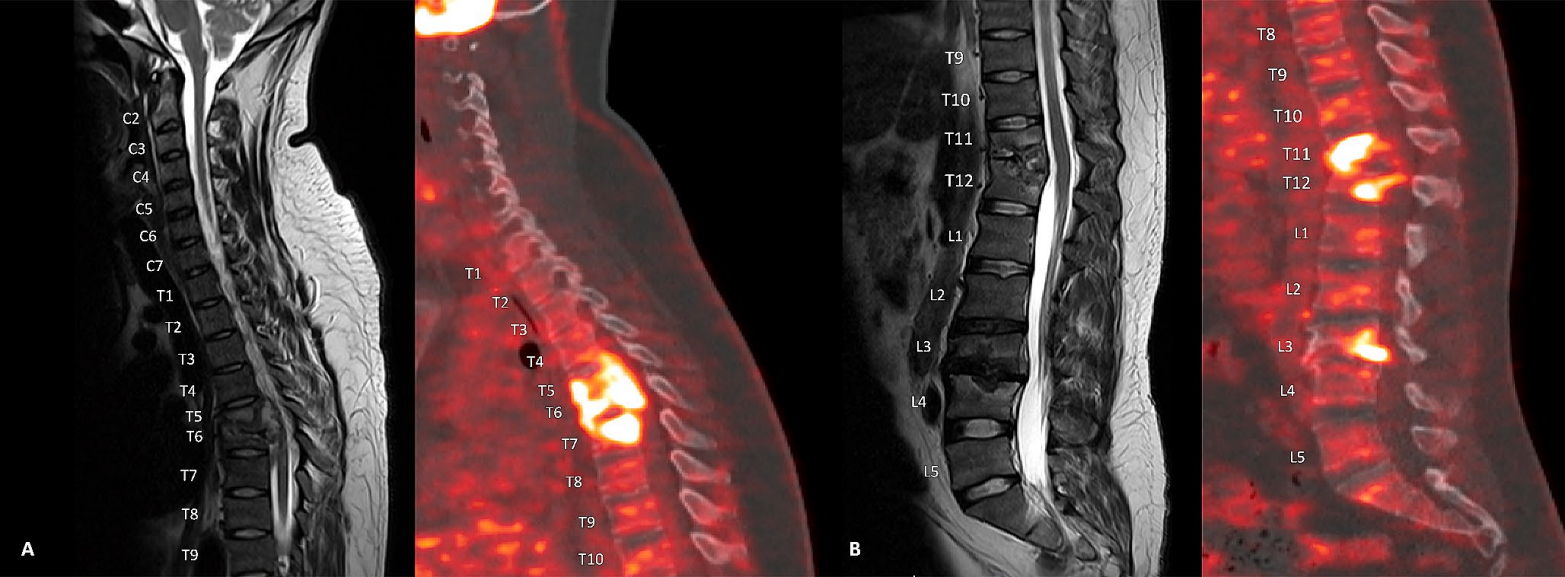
A: Upper thoracic lesion on MRI and 18-FDG-PET/CT on sagittal plane. B: Thoracolumbar lesions on MRI and 18-FDG-PET/CT on sagittal plane
